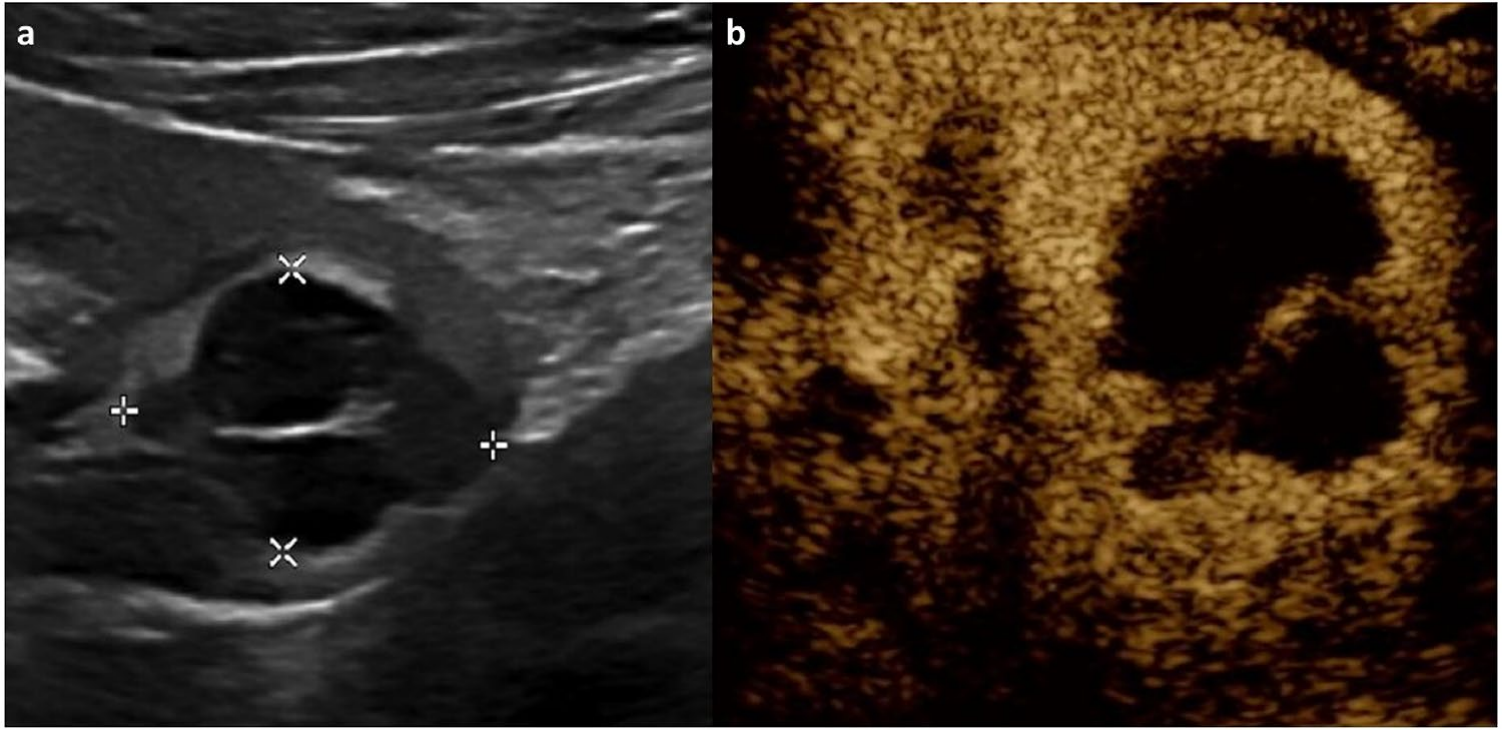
17-year-old male. CEUS study of the left kidney, transverse section. Renal CEUS performed following incidental finding of a renal cyst on CT abdomen and pelvis being performed for jaundice, showing a multiloculated lesion on grayscale (a) with a thin, enhancing septation on CEUS (b) and no solid component, graded IIF
